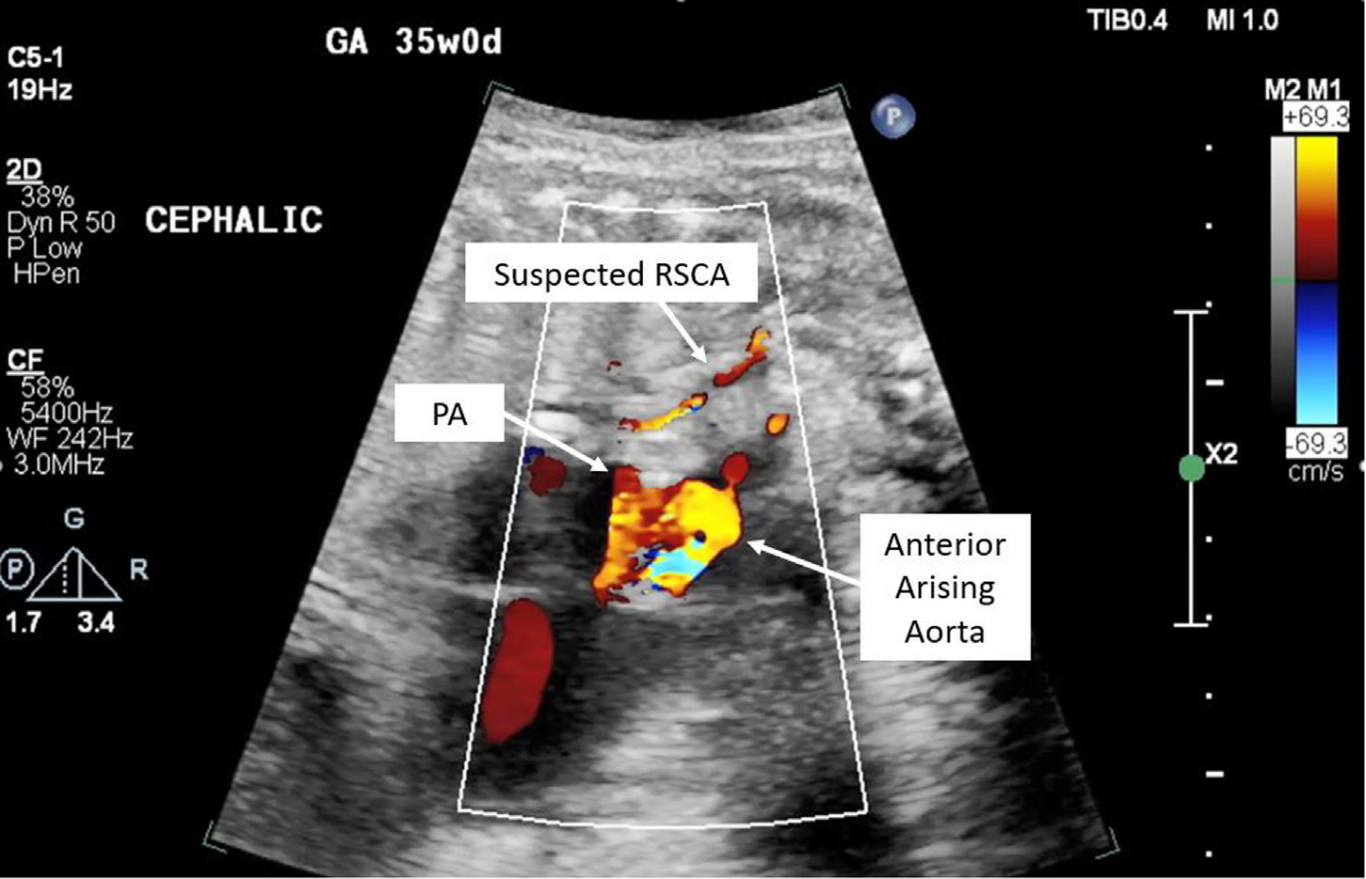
Two-dimensional fetal echo image with color flow Doppler, oblique sagittal orientation, demonstrates anteriorly arising aorta and the suspected RSCA appearing to arise from the PA.
